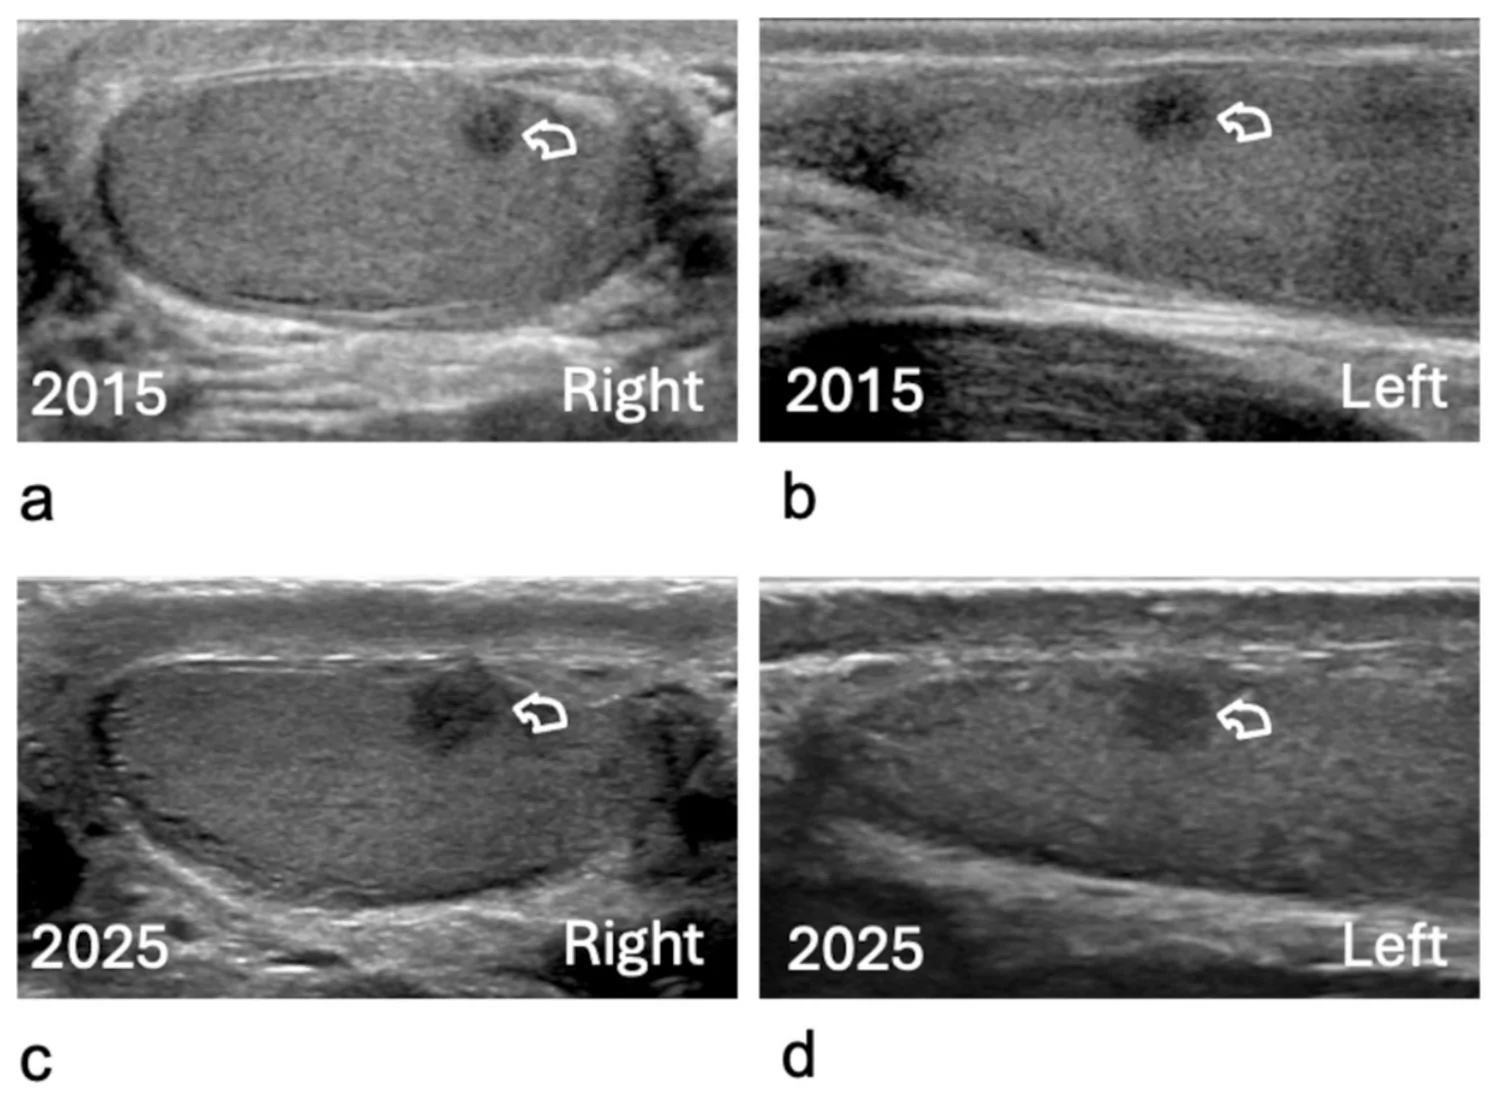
A 40-year-old infertile man. (a,b) Ultrasound shows small testes with two small, non-palpable hypoechoic focal lesions, measuring 3 mm, in the right (a) and left (b) testis, respectively (curved arrows). The patient was managed with imaging follow-up. (c,d) Follow-up examination obtained 10 years later in the same patient shows only a minimal increase in lesion size (curved arrows) to 4 mm, supporting their benign nature.
